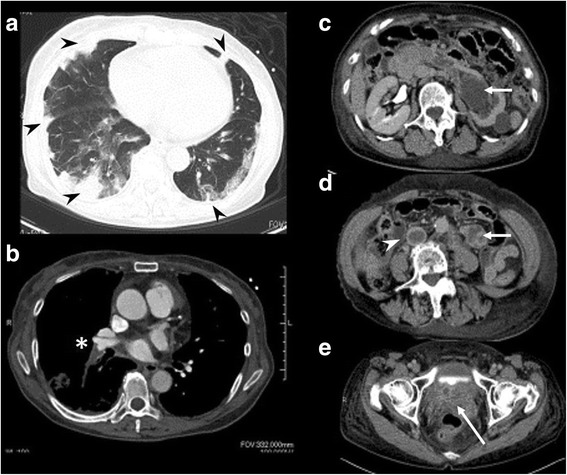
Whole-body computed tomographic images showing several metastatic lesions. The *arrowheads* and *asterisk* indicate tumors. a Multiple nodular and infiltrative shadows were seen mainly in the peripheral lung field. b Contrast-enhanced computed tomography revealed tumor emboli in the right pulmonary artery. c, d Left hydronephrosis indicates involvement of the lymph node near the left urinary duct. There were tumor thrombi in the inferior vena cava leading to the bilateral common iliac vein. e The uterus was completely occupied by the tumor﻿
